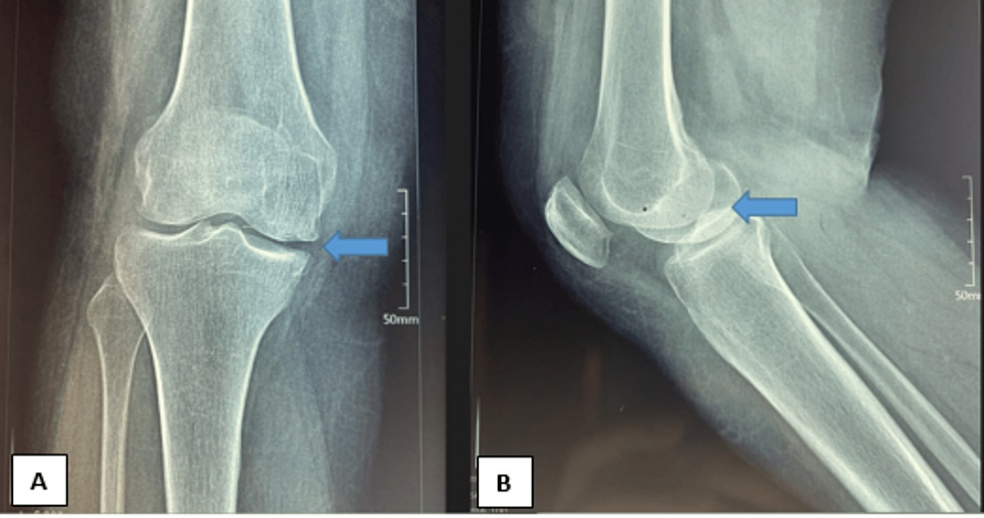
Plain radiographs show degenerative changes on the medial aspect of the knee A: Anteroposterior radiographs of the right knee. B: Lateral radiographs of the right knee
